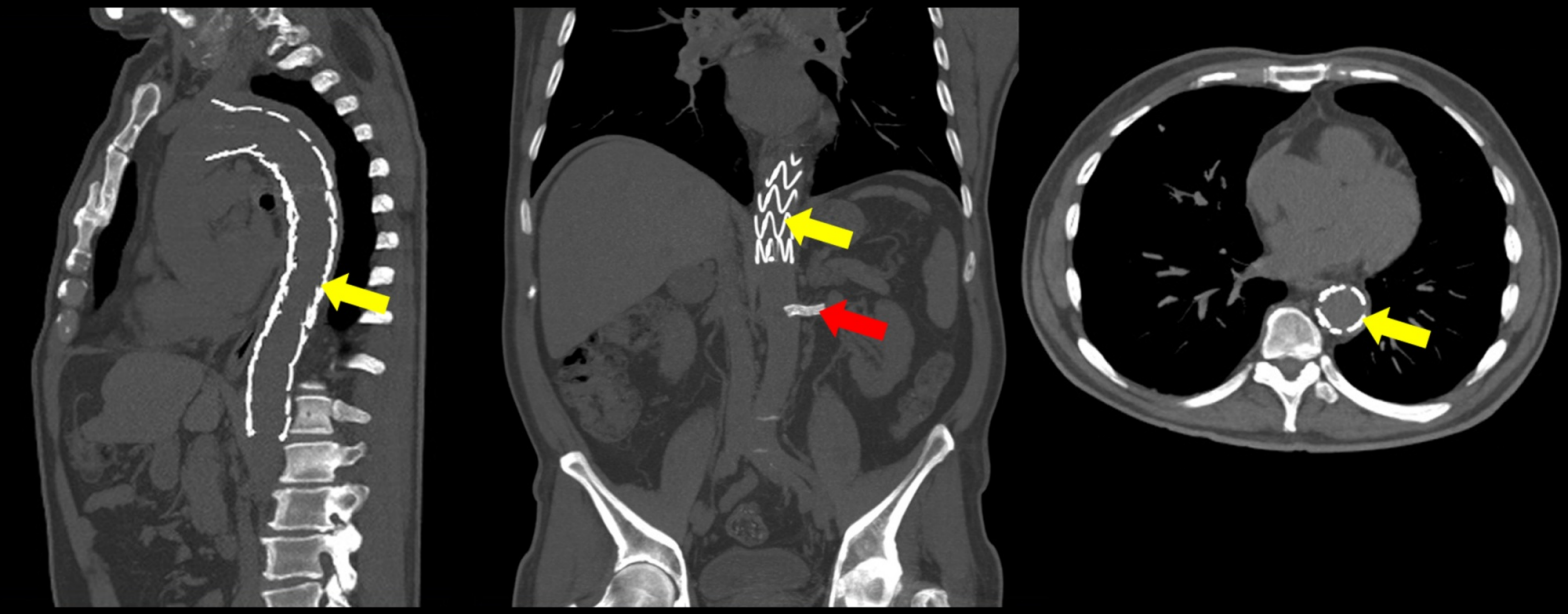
Follow-up sagittal, coronal and axial planes CT showing correct placement of the two Valiant type endoprosthesis after the emergence of the left subclavian artery and right above the celiac trunk, respectively (yellow arrows), and the Viabahn and Assurant stents in the left renal artery (red arrow). CT: computed tomography
